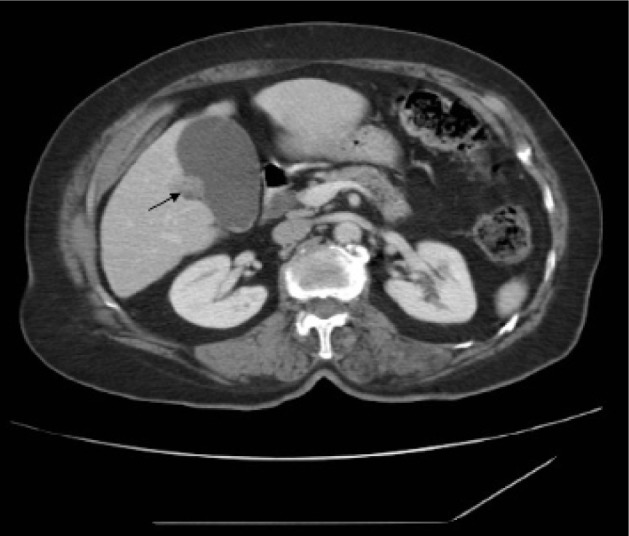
Computed tomography showing small focal lesion (arrow) originally suspected to be a neoplastic lesion
